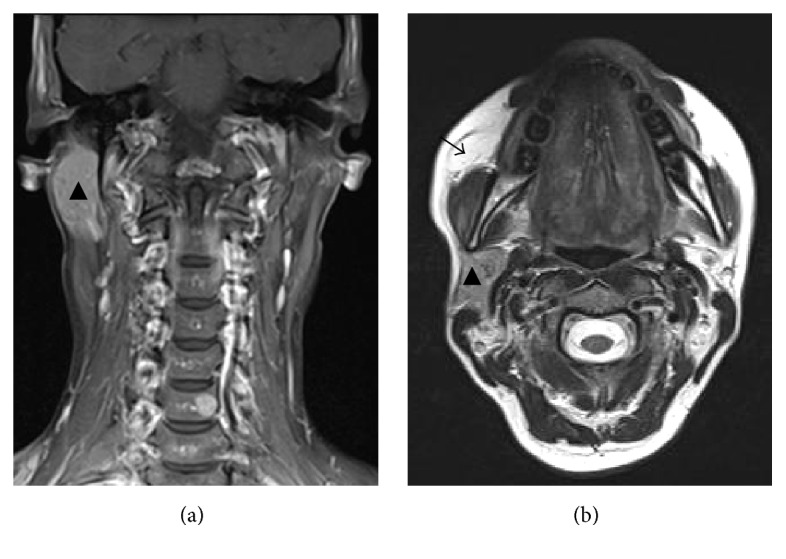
MRI scan of the parotid gland shows the unilateral agenesis of the parotid gland. (a) Coronary scan and (b) axial scan. The arrow points to the lipoma of the cheek and the triangle points to the right parotid gland.
